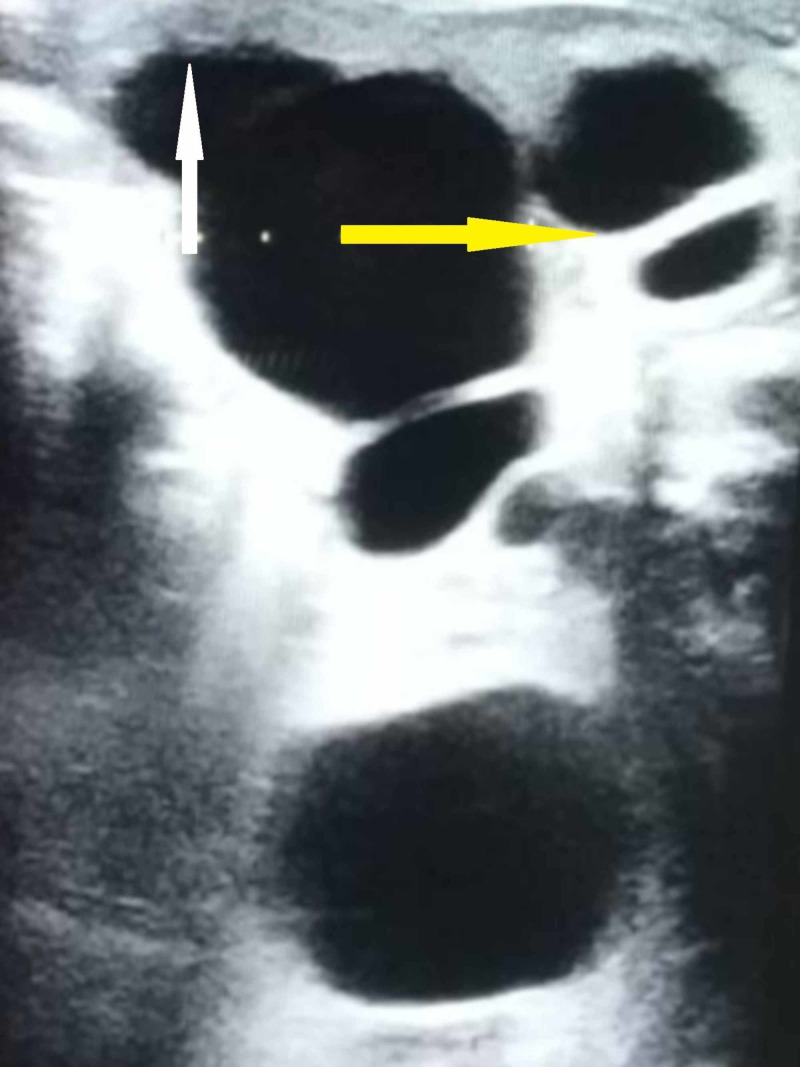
Ultrasonogram image depicting the grossly dilated lower calyx (yellow arrow) with a thin rim of renal parenchyma (white arrow)
